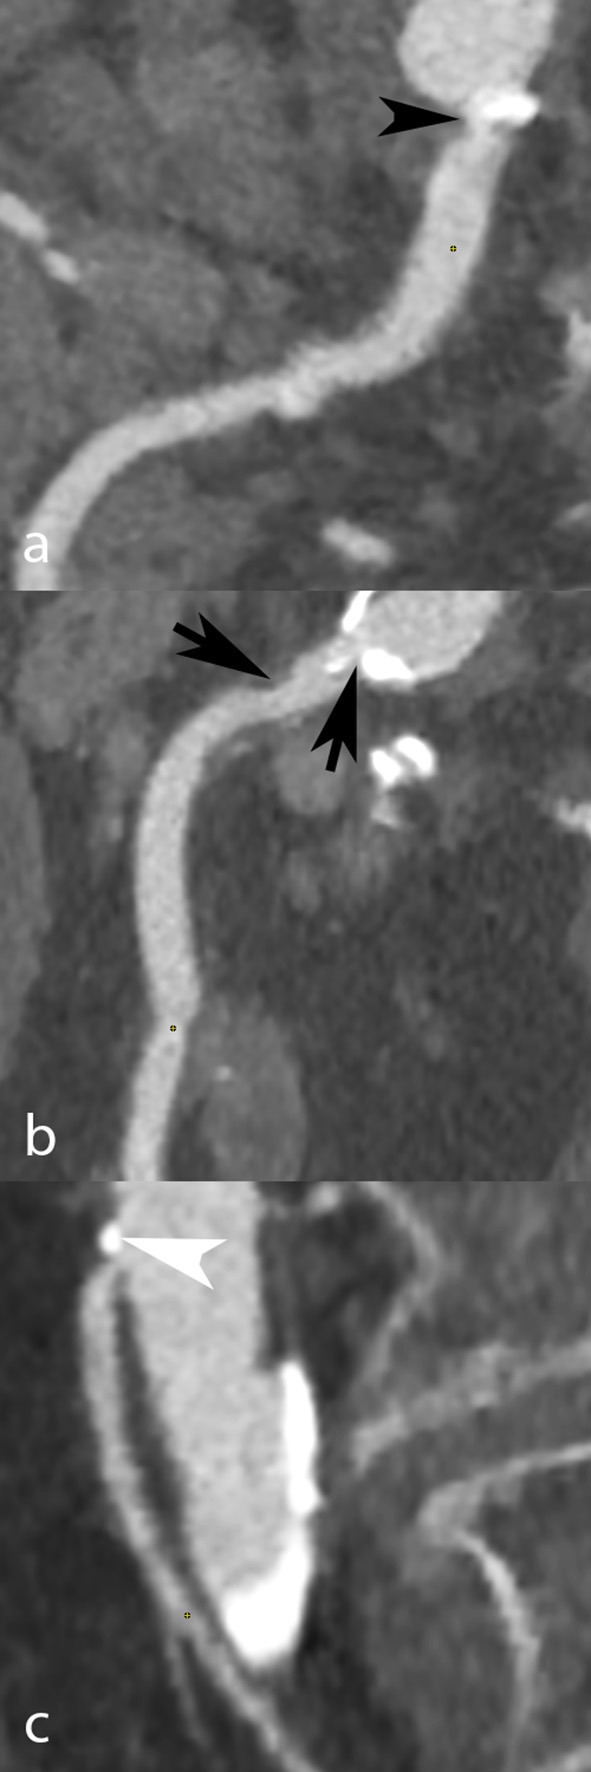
CT angiography in a 90-year-old woman scheduled to undergo TAVI. Curvilinear reconstructions show the coeliac trunk (a), superior (b), and inferior mesenteric artery (c). All three arteries had significant ostial occlusive disease, due to mixed plaques at the coeliac (black arrowhead) and inferior mesenteric (white arrowhead) arteries' ostium, and to a mixed ostial plaque associated with a non-calcified post-ostial plaque (black arrows) in the superior mesenteric artery.
